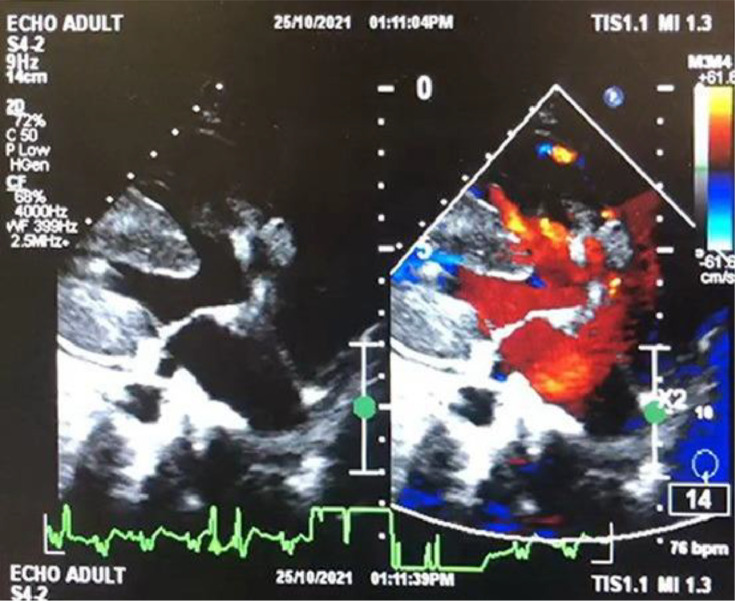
Echocardiography revealed a dilated LA, dilated RA, biventricular hypertrophy, PM VSD 16-19 mm.
